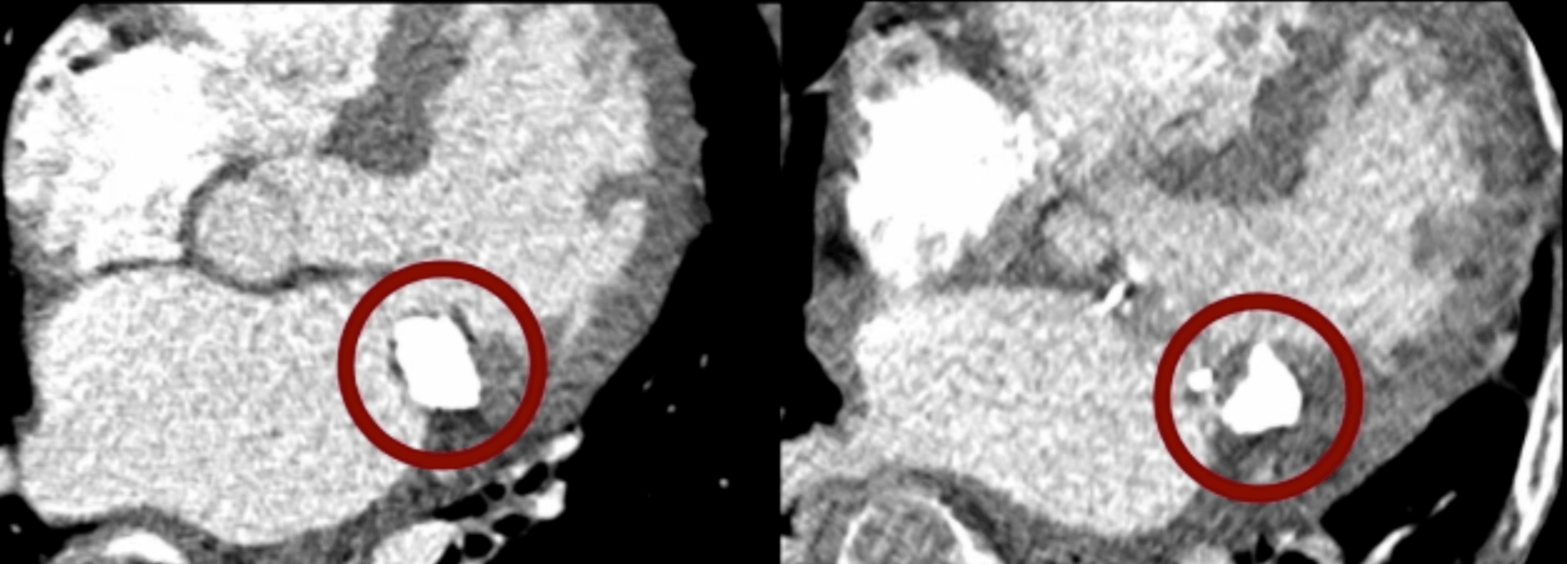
A comparison of pre-operative (left) and post-operative (right) CT scans shows a reduction in the size of the mass following partial resection CT: computed tomography
